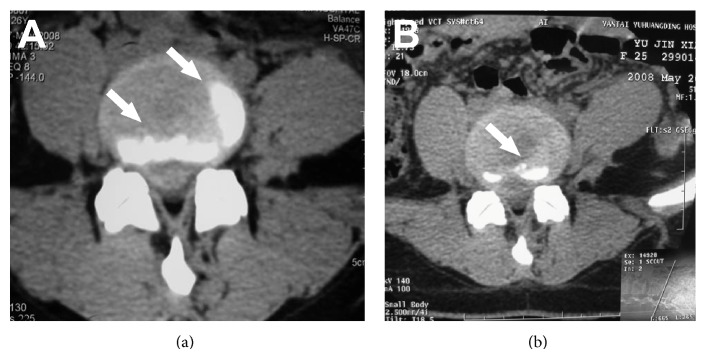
(a) Pre- and (b) postoperative CT images of a representative patient with L4-5 intervertebral disc herniation (the protrusion is indicated by white arrows); protrusion was reduced significantly 1 week after the operation (as indicated by white arrows).
